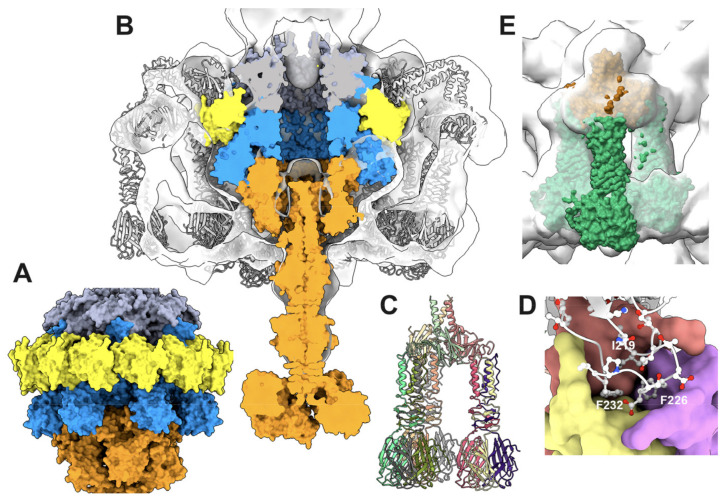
The peripheral baseplate. (A) Surface view of the dodecameric BppU N-terminal domain (yellow) complexed to the hexameric Dit (blue): MTP: gray; Tal: orange. (B) Split view of the nsEM map (EMD-1793; 25 Å resolution) of the complete baseplate with the distal hexameric MTP (gray), the hexameric Dit (blue), the trimeric Tal (orange), and the dodecameric BppU N-terminal domain (yellow) fitted inside. The gray ribbon inside the map represents the X-ray structure (PDB id 4v96). (C) The AF2-predicted structure of the “tripod”, formed of the trimeric BppU C-terminal domain holding three RBP trimers. (D) Close-up of the BppU/RBP interface with the hydrophobic residues Ile219, Phe226, and Phe232 also identified in the X-ray structure. (E) Surface view of the “tripod” fit in the nsEM map.
